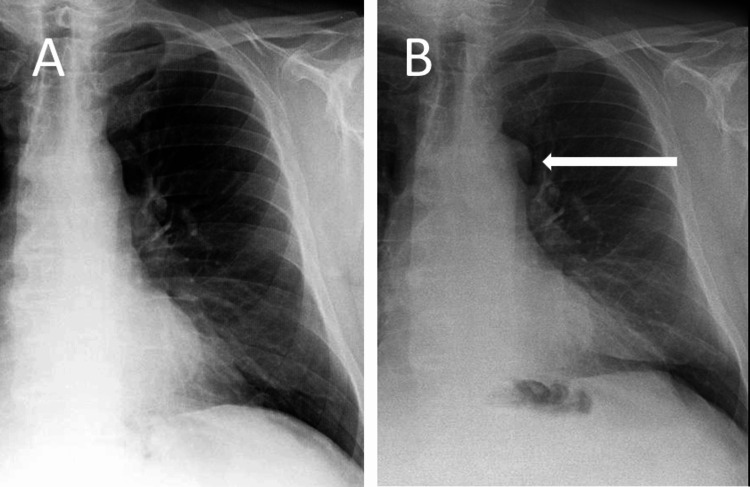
Posteroanterior chest radiographs of the patient at two different time points Panel A shows the normal chest radiograph of the patient without a double aortic contour. Panel B shows a double aortic contour (arrow) due to the lymphadenopathy.
